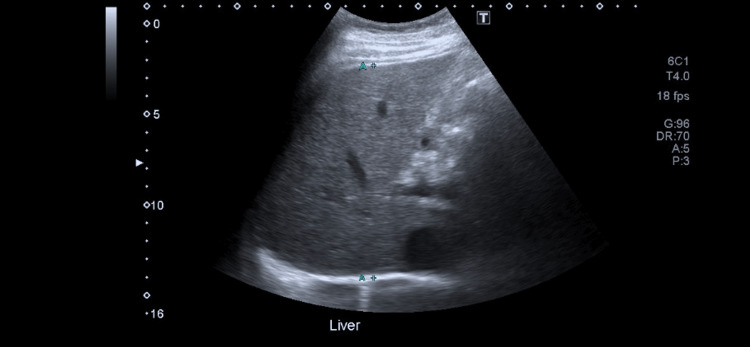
Ultrasound showing the liver.
